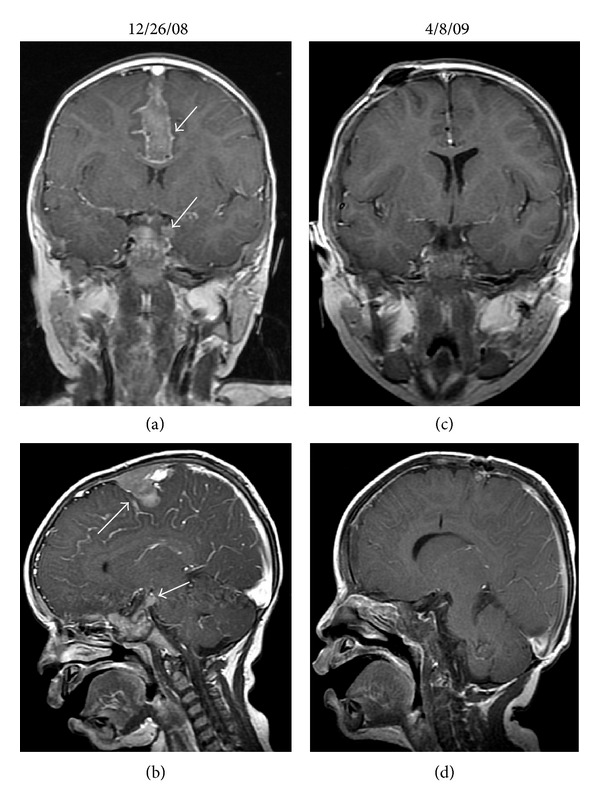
(a) 12/26/08 MRI coronal T1 postgadolinium image demonstrates a dural-based mass along the falx with heterogeneous enhancement (arrow). (b) 12/26/08 MRI sagittal T1 post-gadolinium image demonstrates the mass along the falx and an enhancing focus in the suprasellar cistern (arrows). (c) 4/8/09 MRI coronal T1 post-gadolinium image shows resolution of the previously identified enhancing mass within the falx. An Ommaya reservoir has been placed in the interim. (d) 4/8/09 MRI sagittal T1 post-gadolinium image shows resolution of previously seen enhancing anterior falx and resolution of the suprasellar mass.
